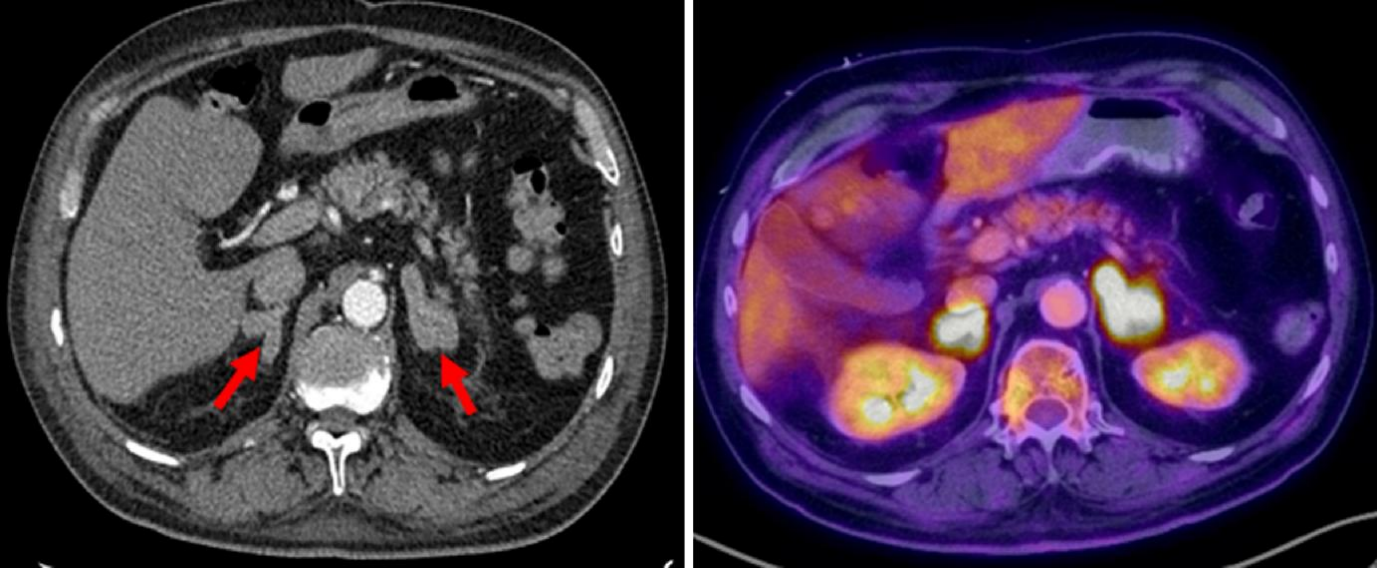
Bilateral adrenal glands thickening without discrete nodules on CT abdomen/pelvis (left) and PET/CT (indicated by red arrows).
